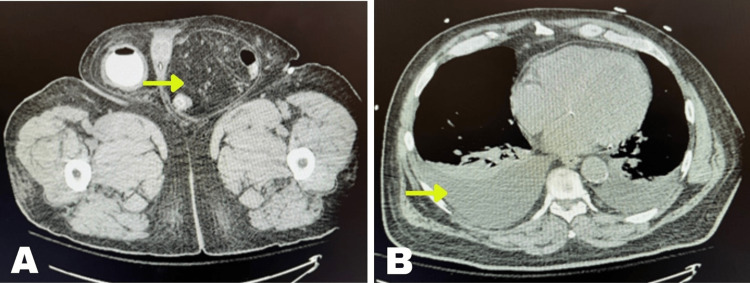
(A) Abdominal CT displaying a massive left inguinal hernia; (B) Chest CT scan featuring pulmonary edema CT, computed tomography
